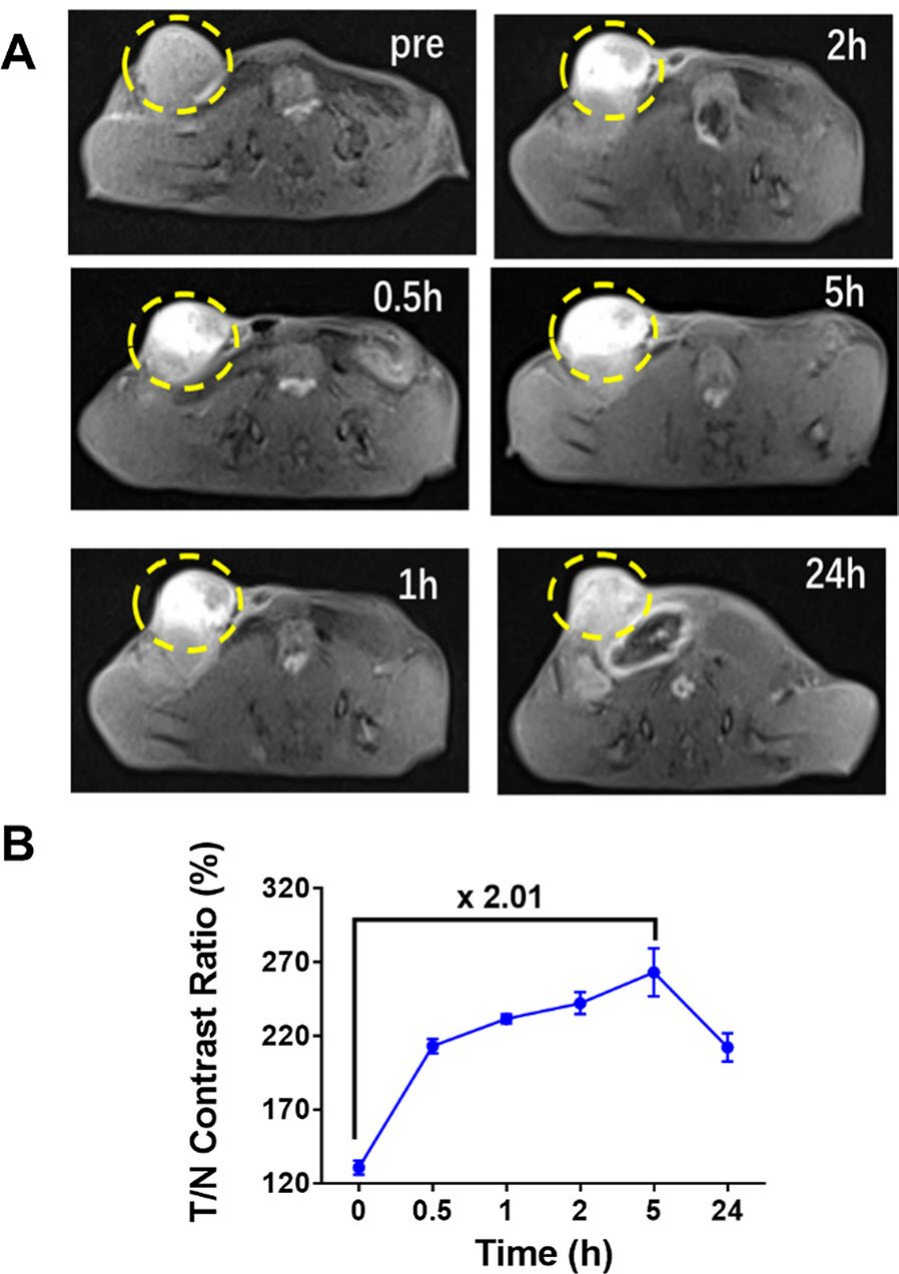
MRI imaging (T1WI) of 4T1 orthotopic tumor model after intratumoral injection of MnP@Lip (n = 3, Mn 1 mg/kg). A The representative MR images of tumor. The yellow circles indicate the location of the tumor. B The quantitative results of MRI signal intensity in tumor
